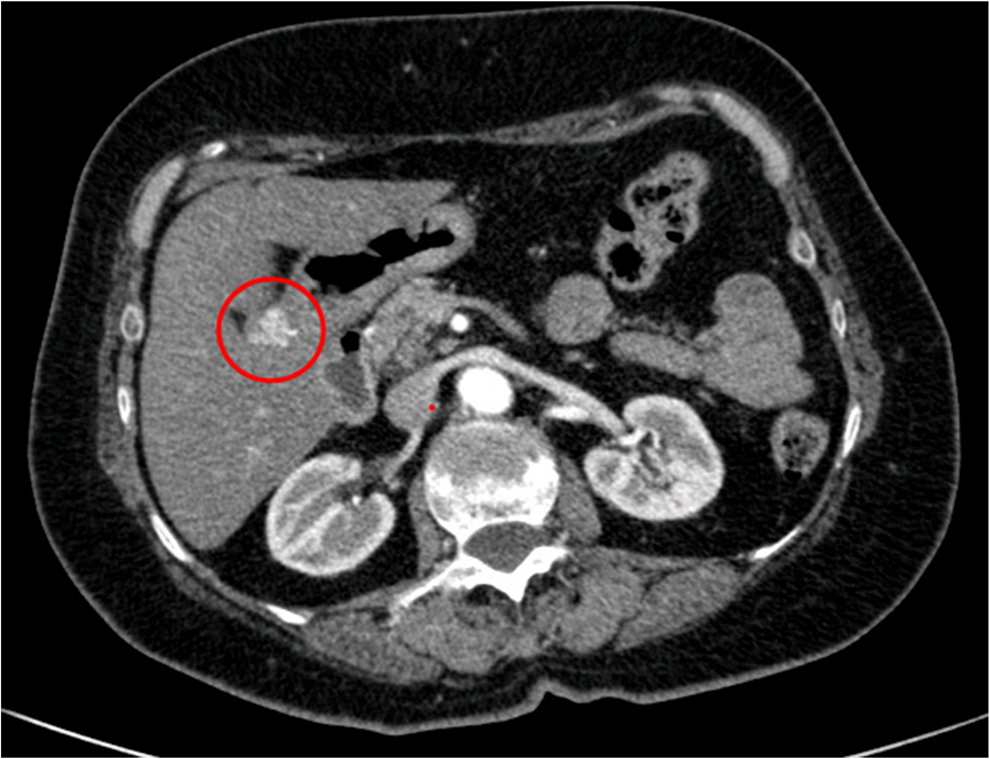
Computed tomography. Enhancement of the lesion in arterial phase that shows the lesion within the gastric wall
